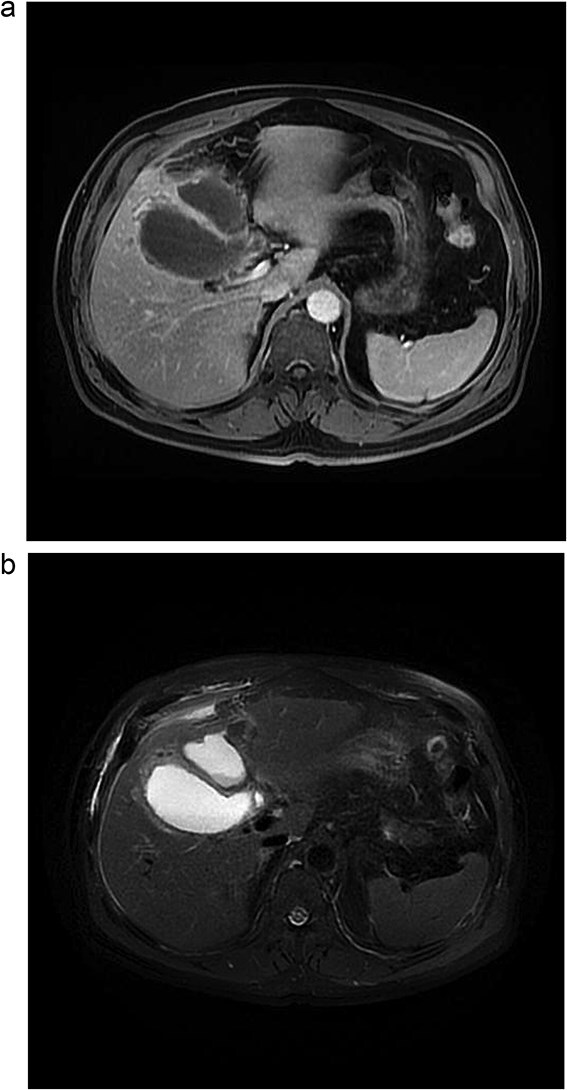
Abdominal MRI. (a) Axial 3D T1 LAVA-Flex *water-only* sequence, venous phase. (b) Axial T2 PROPELLER sequence with fat suppression.
